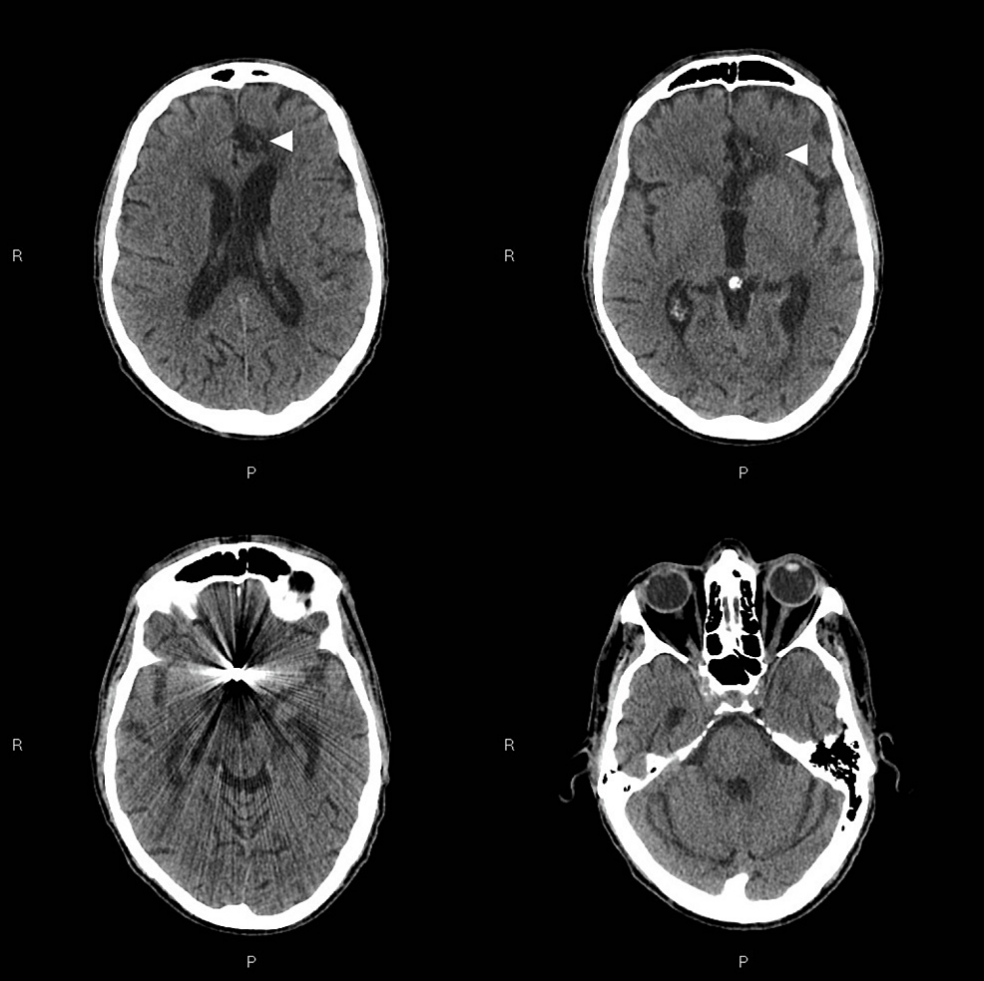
Six-month follow-up computed tomography (CT) scan after a diffuse subarachnoid haemorrhage due to rupture of an anterior communicating artery (AcomA) aneurysm The patient experienced a diffuse subarachnoid haemorrhage due to rupture of an anterior communicating artery (AcomA) aneurysm then treated by endovascular deposition of platinum coils. Six-month follow-up computed tomography scan demonstrated regular dimension of the ventricular system and a malacic transformation of an intraparenchymal hematoma in the medial aspect of the left frontal lobe (white arrowheads)
